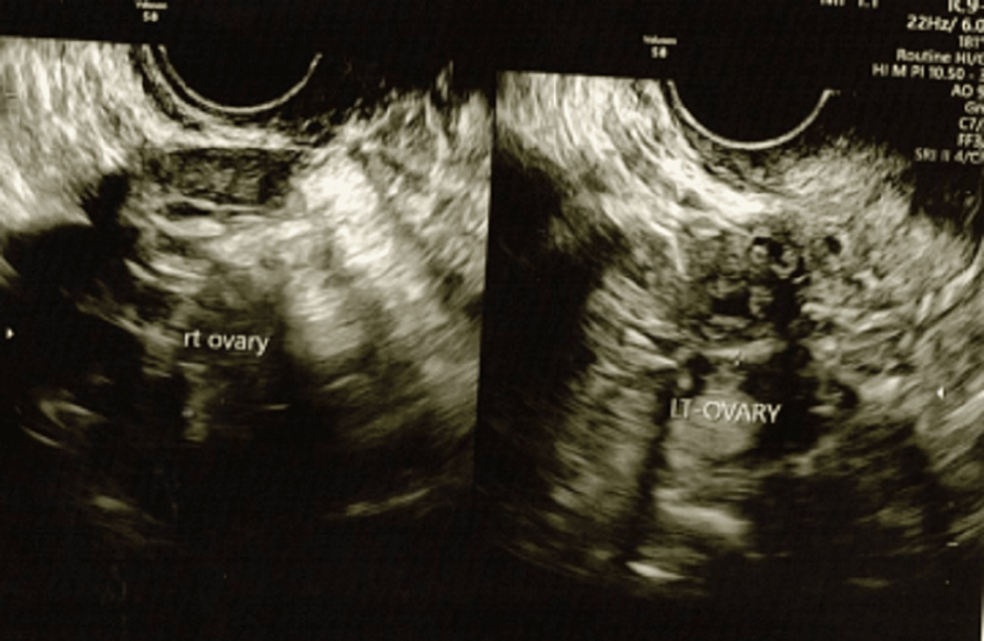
Ultrasound of the ovaries
